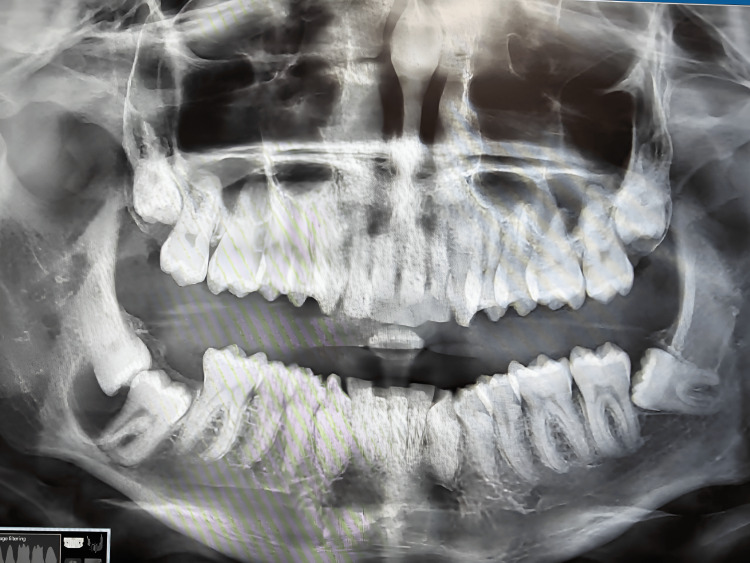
OPG revealed a unilocular radiolucency surrounding the crown of the impacted 48 OPG: Orthopantomogram
